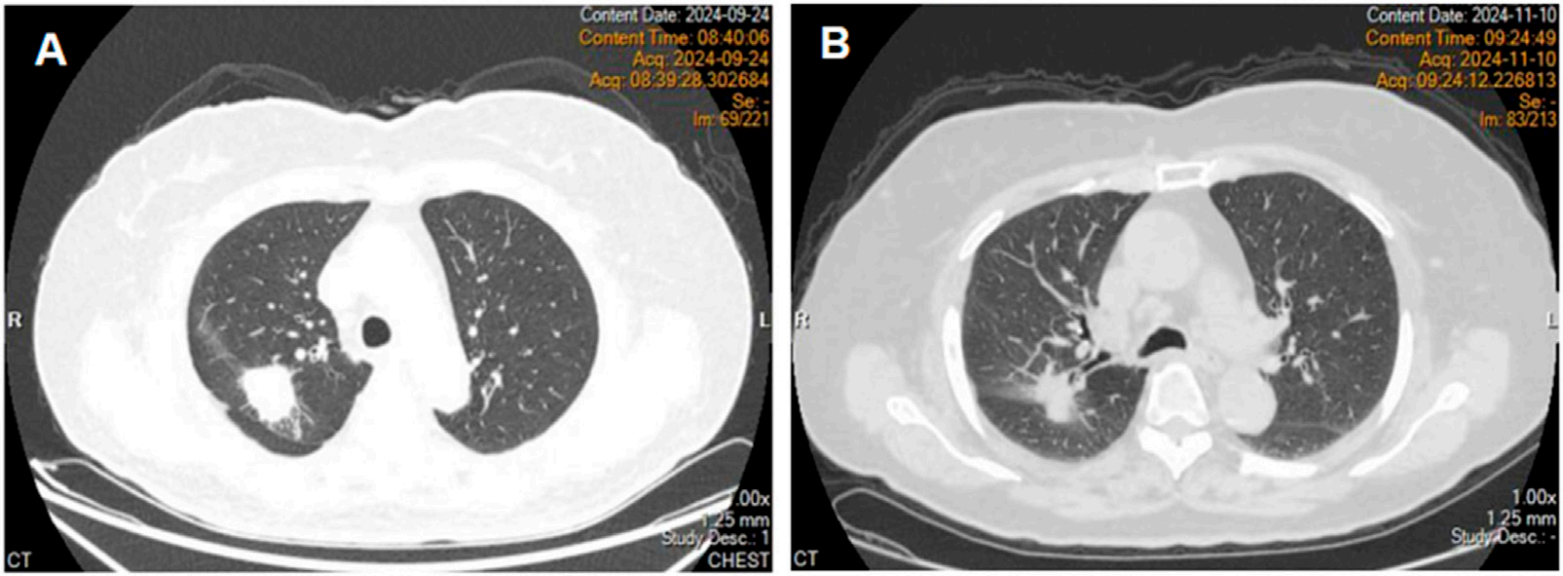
Chest computed tomography revealed a right upper lobe mass (A). Chest computed tomography on day 31 of treatment (B).
